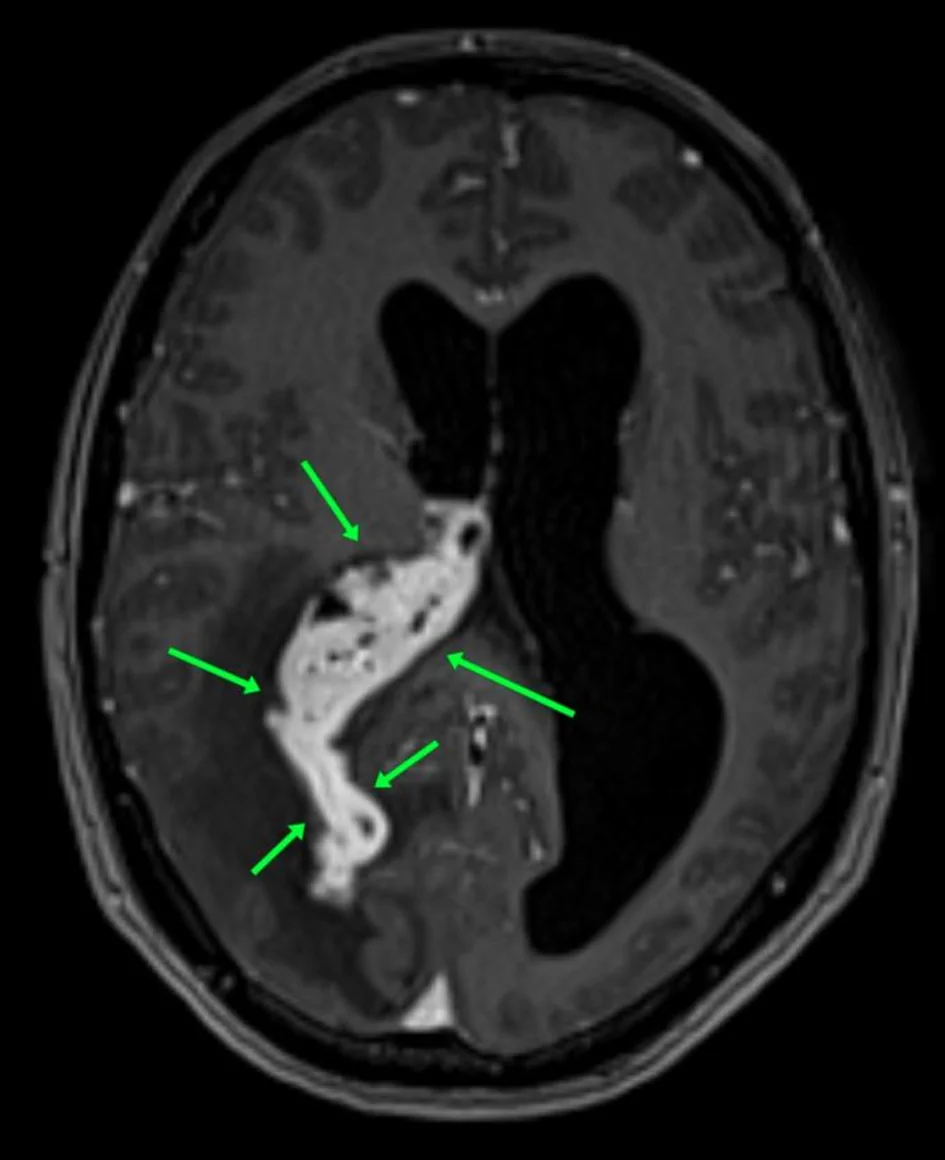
Post-contrast axial T1-weighted MRI demonstrating intense, heterogeneous enhancement of the lesion (green arrows). MRI: magnetic resonance imaging.
